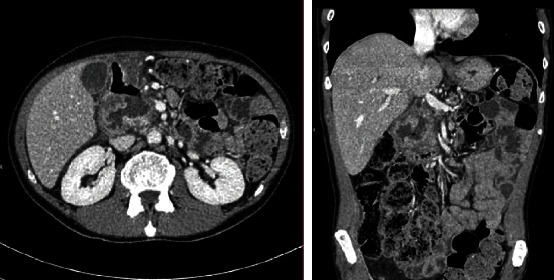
Computed tomography findings: parietal thickening affecting the antropyloric region and first duodenal portion with an ulcerated appearance as well as trabeculation of the adjacent fat and the presence of adenopathies, initially interpreted as suspicious of a neoformative nature.
